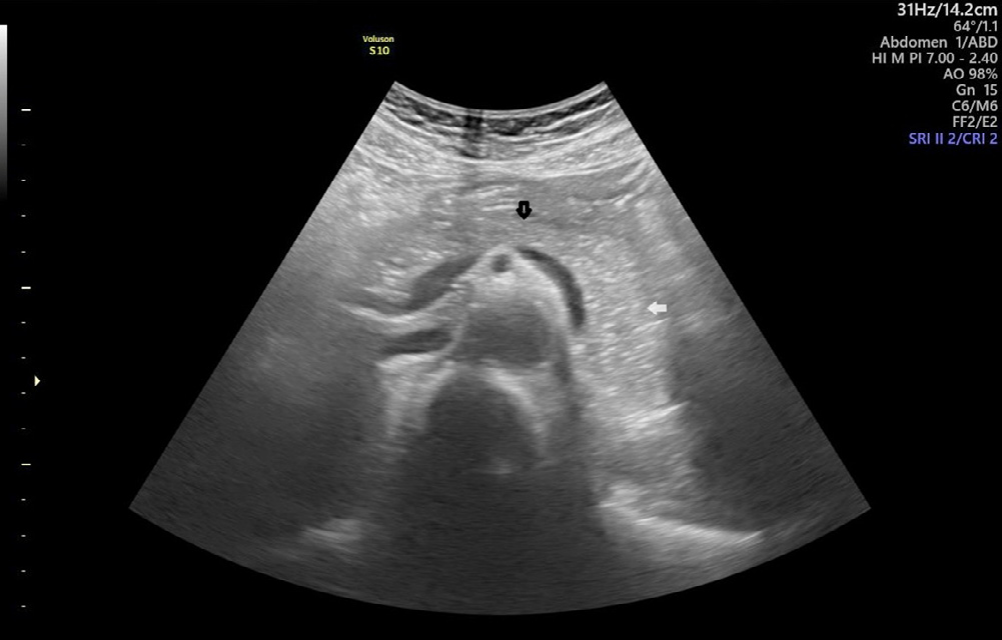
Ultrasound image of the pancreas, with a focused scan on the body and tail region. No hypoechoic or hyperechoic abnormal lesions were detected in the pancreatic body (black arrow) or tail (white arrow).
